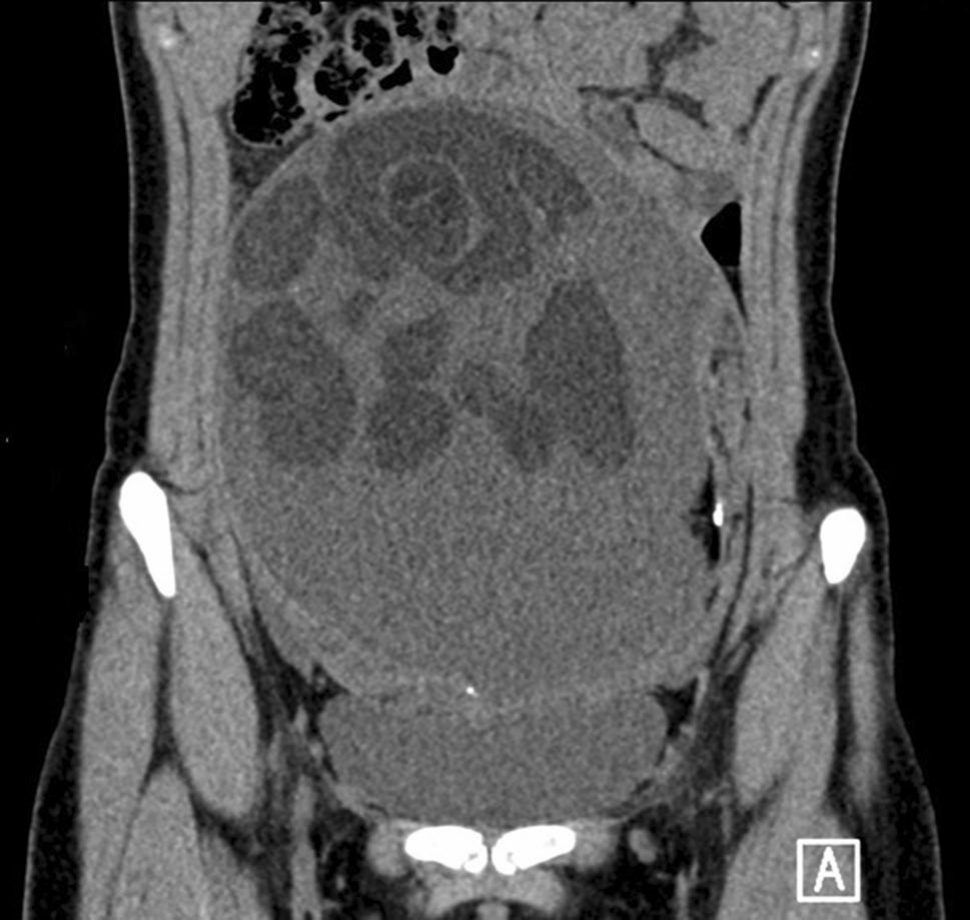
Mature ovarian teratoma with numerous floating balls visualized by computed tomography of a 16-year-old patient
